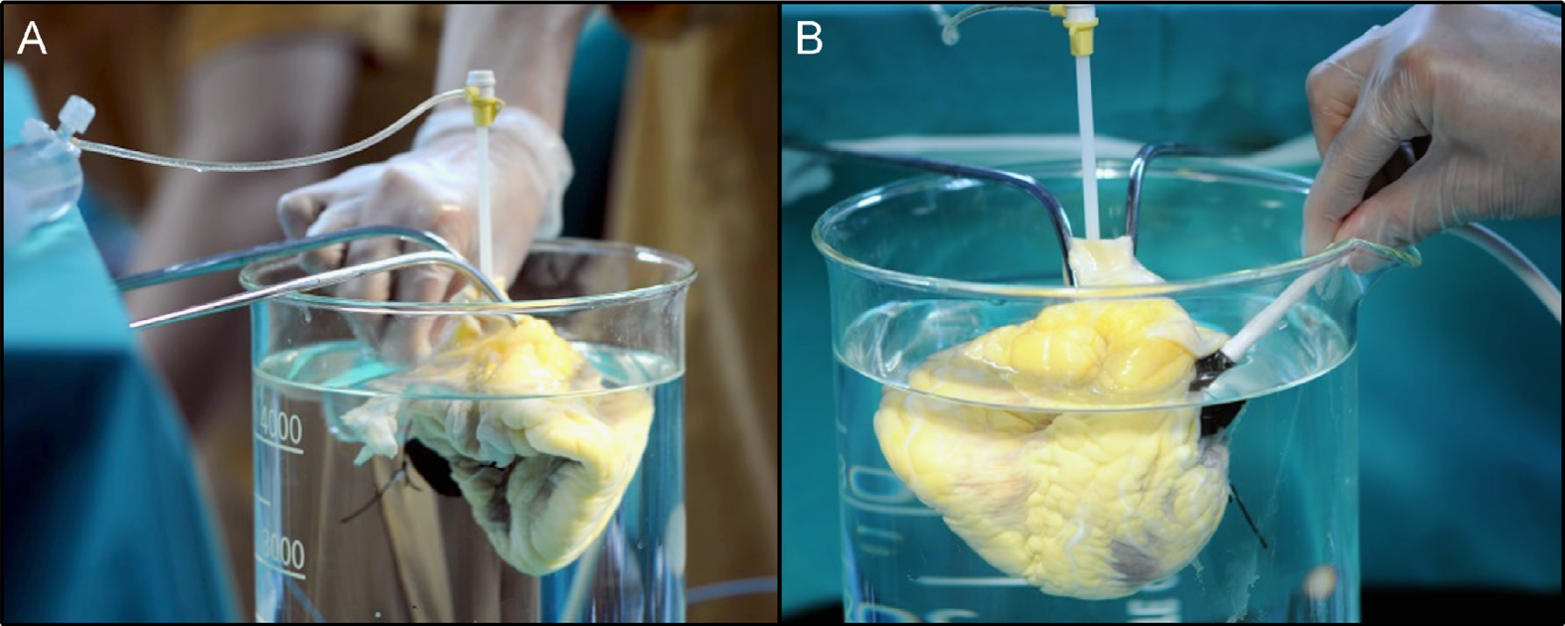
Valve competence evaluation during systole and diastole using echocardiography from the epicardium.
